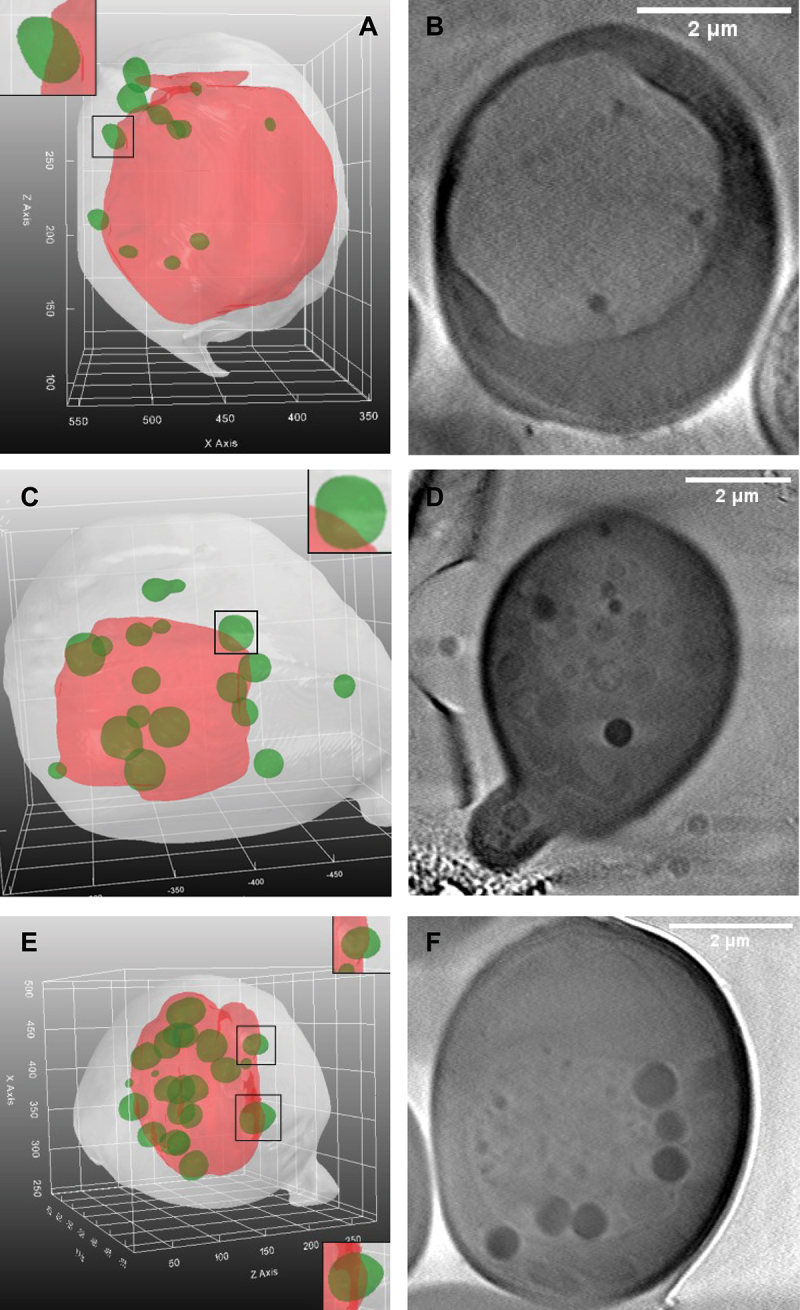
Vacuolar shapes from 3D renderings for wild-type cells and for yeast lacking either Ncr1 or Npc2. 3D renderings as obtained from the segmentation CNN model for wild-type cells (A), *ncr1∆* cells (C) and *npc2∆* cells (E). Insets show zoomed versions of rectangular black boxes highlighting green LDs docking on the surface of the vacuole, shown in red. Corresponding10 slice sum projections for X-ray reconstructions from corresponding tomograms for wild-type cells (B, *n* = 20), *ncr1∆* cells (D, *n* = 11) and *npc2∆* cells (F, *n* = 5). See main text for further details.
